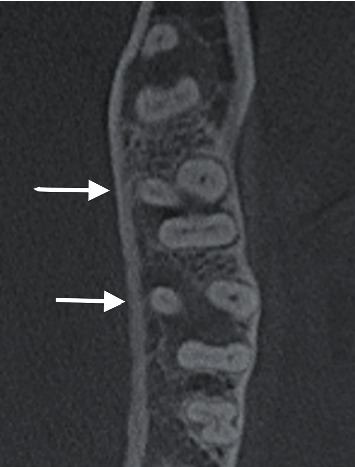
CBCT axial section at midroots of first and second mandibular molars showing the presence of a third root (arrows).
